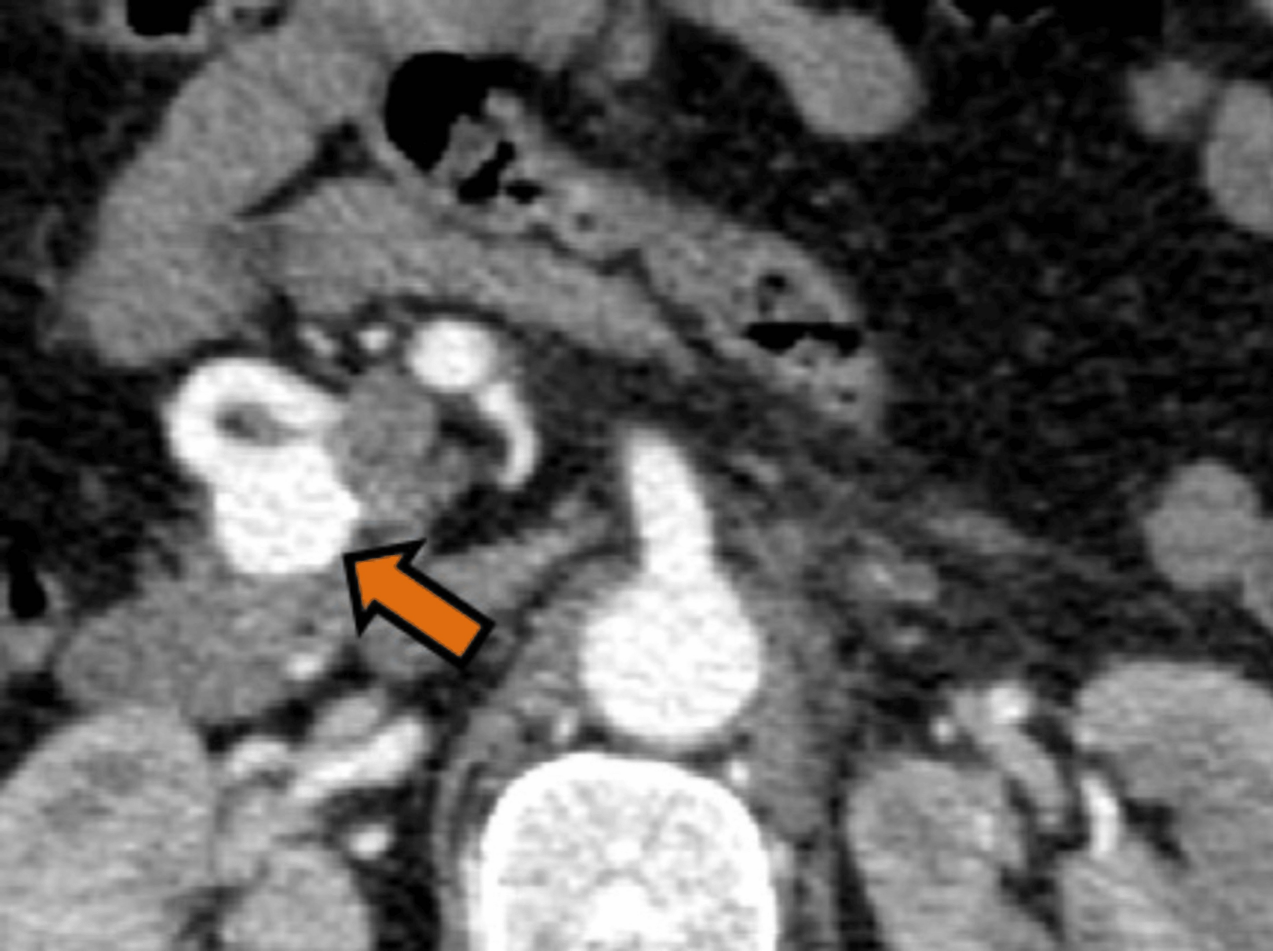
CECT of a 62-year-old trauma victim with incidentally diagnosed AOB aneurysm (Case 2) Axial section of arterial phase shows an aneurysm with few eccentric wall calcifications noted in close proximity along the course of SMA (orange arrow) in the head of the pancreas region CECT: contrast-enhanced computed tomography; SMA: superior mesenteric artery
